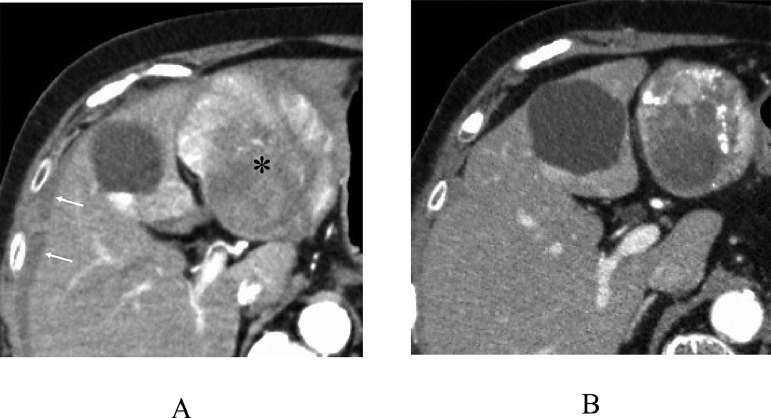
Enhanced computed tomography (CT) of the liver. (A) Enhanced CT just after stabilization of the patient's hemodynamics showed an oval mass, 83 mm in size (asterisk), with heterogenous enhancement and small amount of perihepatic fluid (arrows). (B) Enhanced CT 5 weeks after trans-arterial chemo-embolization showed an oval mass, 61 mm in size, with intratumoral blood flow.
